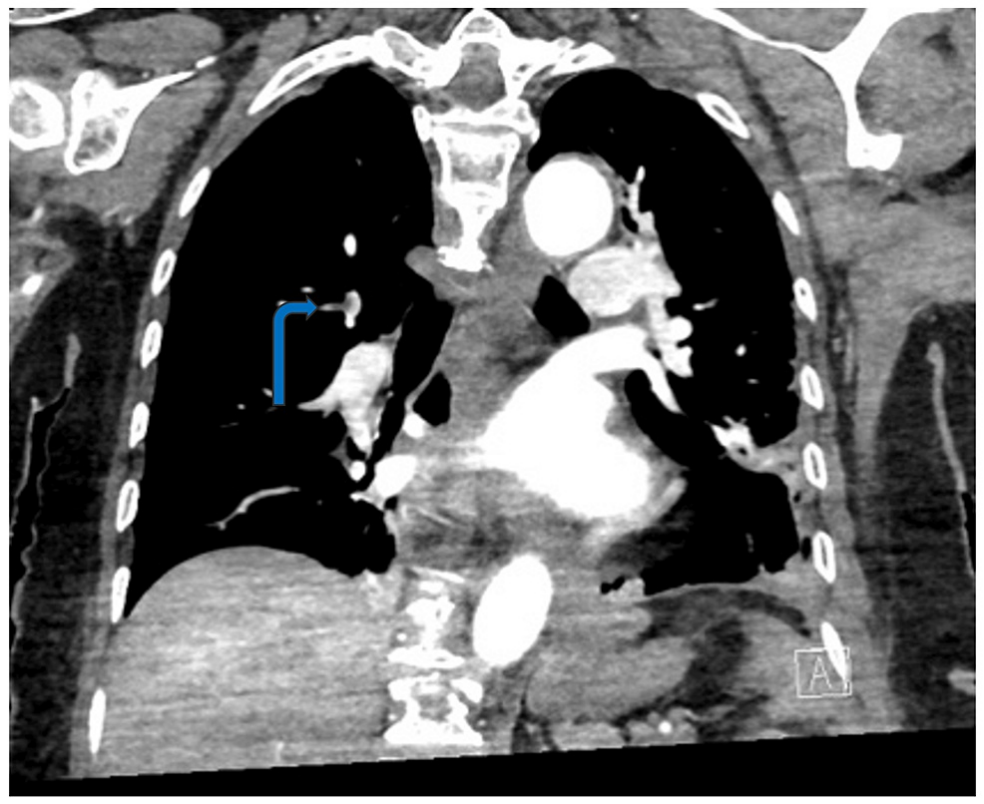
Coronal CT pulmonary angiogram image showing right upper lobe fat embolus extending from segmental to subsegmental branch (arrow).
